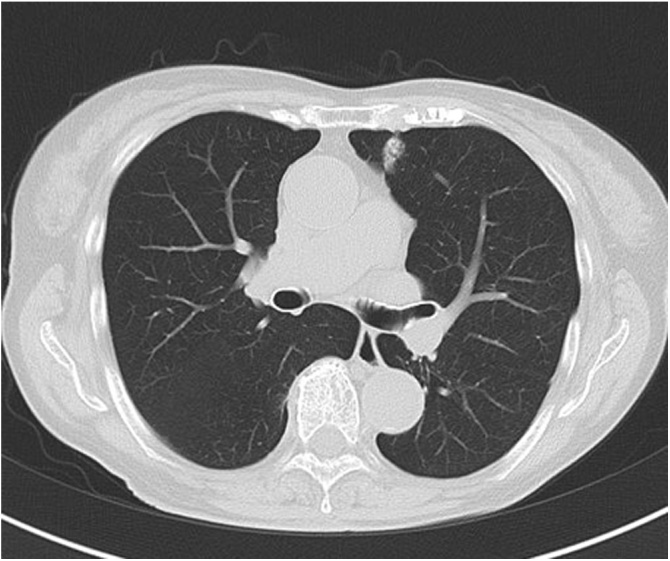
Chest computed tomography revealed a 1.9 × 1.1 cm nodule in the left anterior segment. The nodule was located near the lingular segment.
